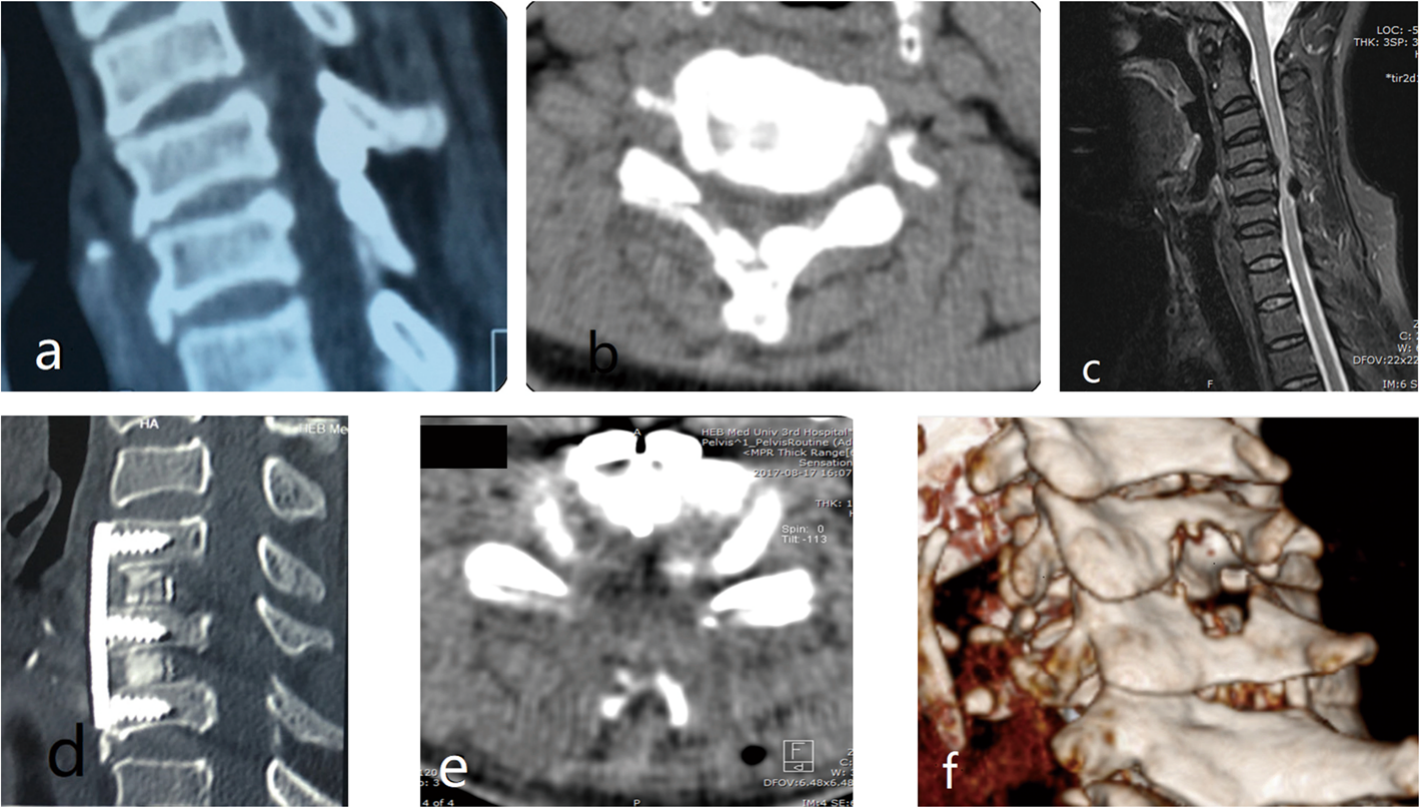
Preoperative and Postoperative Imaging Comparison. Preoperative cervical CT and MRI (a, b, c) showed C4–5 and C5–6 disc herniation with calcification of C5 posterior ligamentum flavum, spinal stenosis and spinal cord compression. In the first stage, anterior cervical decompression and fusion were performed, and the clinical symptoms were not improved thoroughly. In the second stage, bilateral lamina decompression was performed under endoscopy to remove the compression from C5–6 posterior (d, e, f).
